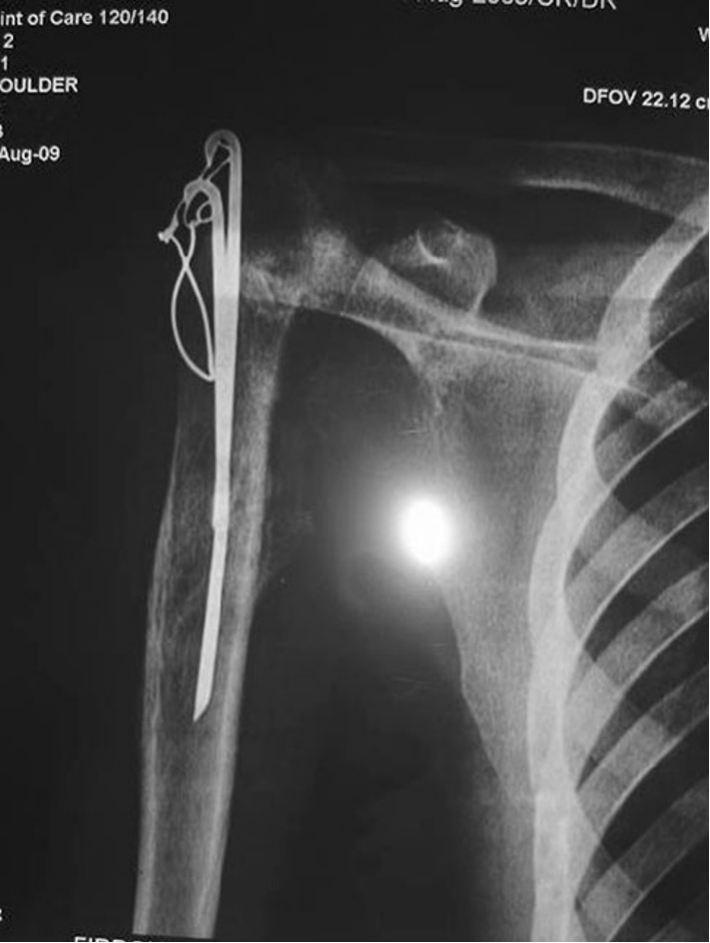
Radiograph 1 year after surgery
